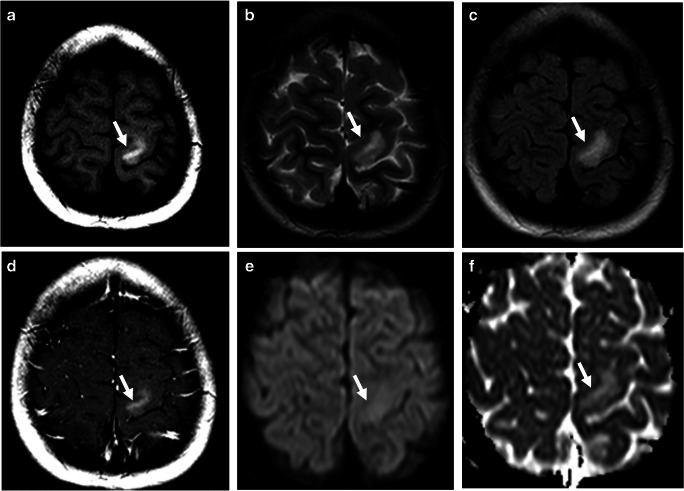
Angiocentric glioma. The tumor (arrows) is hyperintense on T1-weighted (a), T2-weighted (b), and T2 FLAIR (c) images, without appreciable enhancement on the post-contrast T1-weighted image (d). Also, there is no diffusion restriction as seen on the diffusion-weighted image (e) and ADC map (f)
